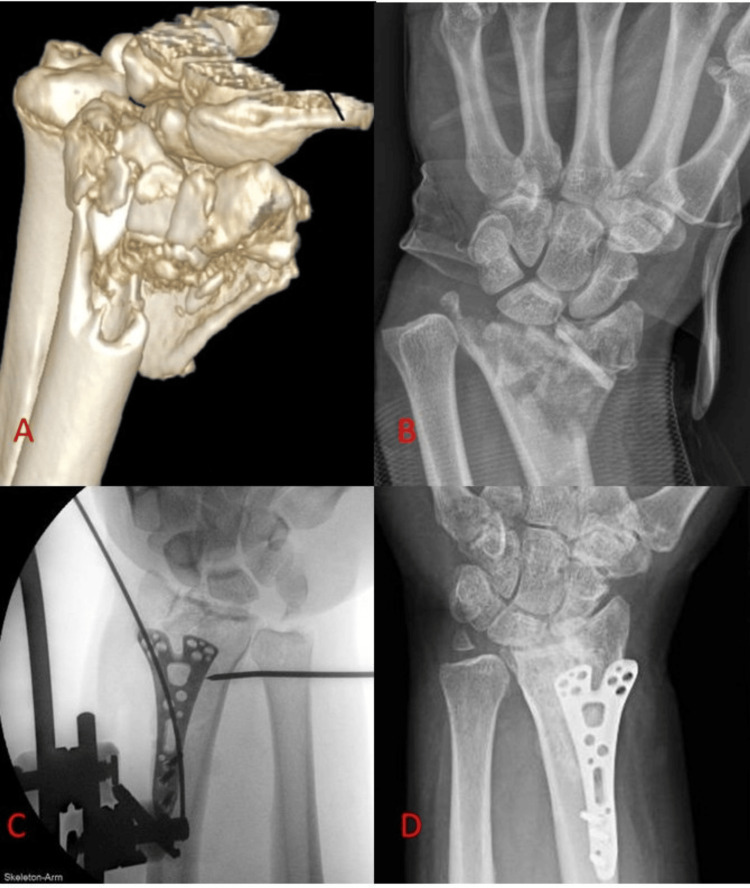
Case example of complex distal radius fracture, which was treated with combined external and internal fixation. (A) CT reconstruction of a highly comminuted fracture of the distal radius, not amenable to volar locking plate fixation. (B) Preoperative anteroposterior radiograph of the same fracture, which was subsequently stabilised with a combination of suture fixation, external fixation, and volar plate fixation. (C) Intraoperative anteroposterior radiograph demonstrating restoration of radial height with external fixator. (D) Postoperative anteroposterior radiograph demonstrating fracture union. The volar plate was removed subsequently.
